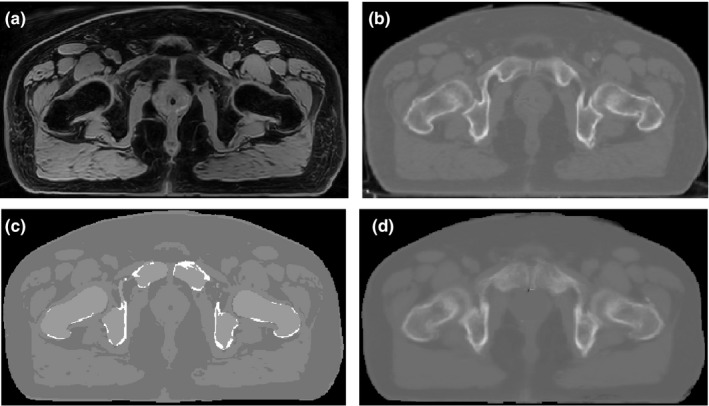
Example of a water‐only image (a) deformed planning CT (b) MRCAT CT (c), and synthetic CT (d) for a typical patient in the test dataset.
